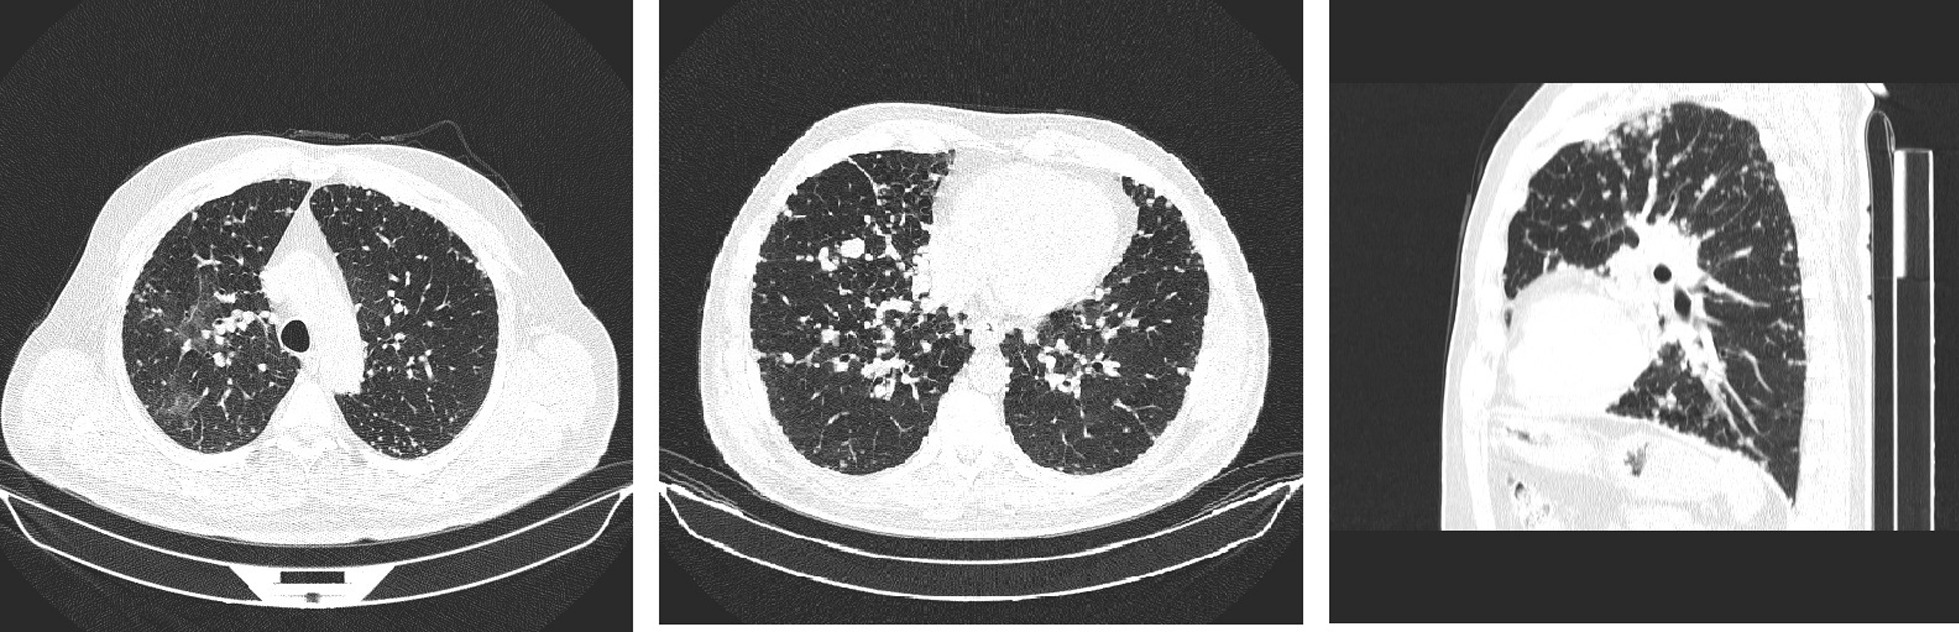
Axial and sagittal views of chest CT scan of a patient with COVID-19 and pulmonary metastasis show nodules and grand glass opacities
